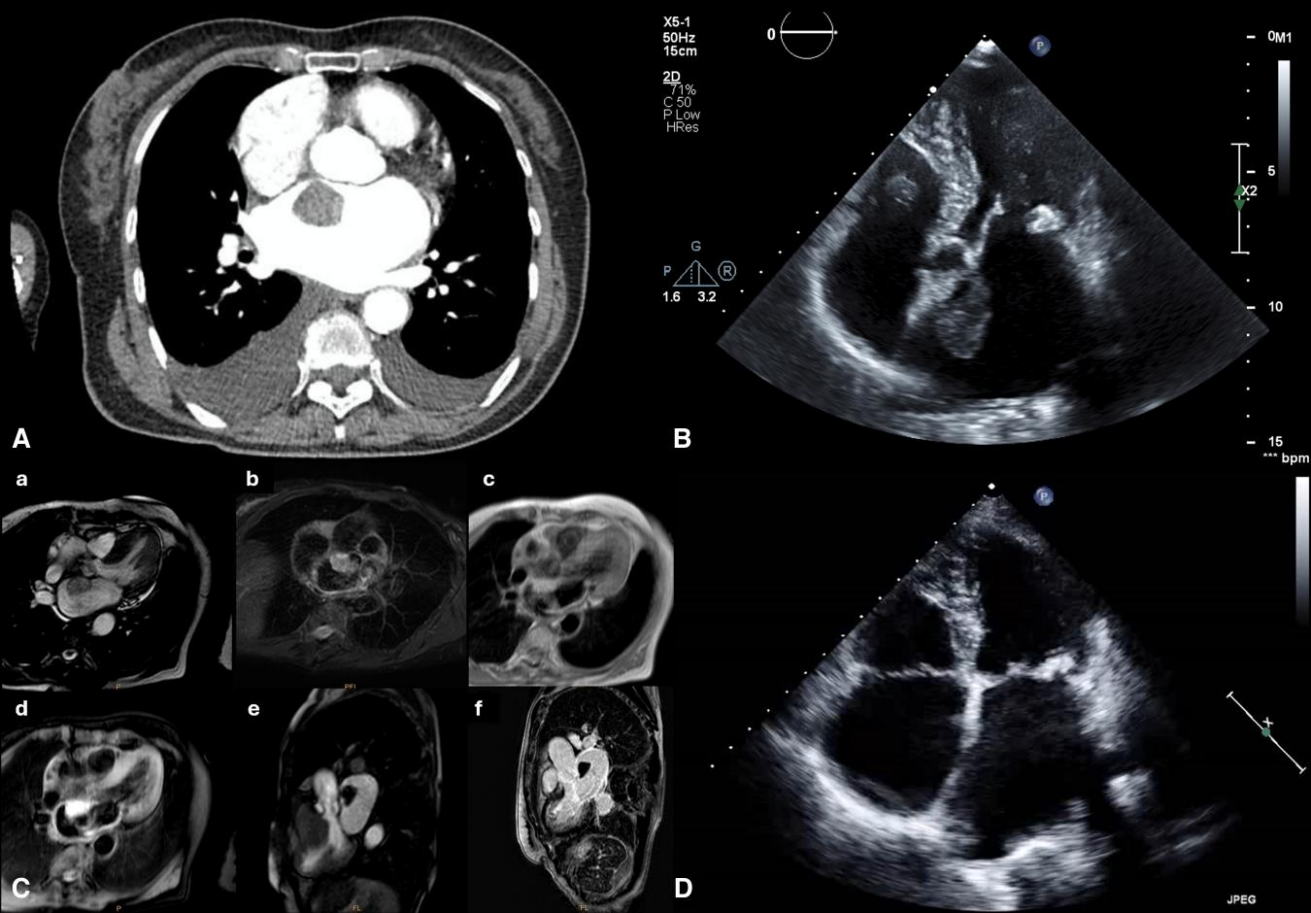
(*A*) Computed tomography (CT) pulmonary angiography demonstrating a large mass in the left atrium; (*B*) Transthoracic echocardiography (5-chamber view) revealing a mobile, heterogeneous mass (30 × 20 mm), attached to the interatrial septum. This image corresponds to Supplementary material online, Videos S1-S2; (*C*) Cardiac magnetic resonance: (C-a) Cine image SSFP sequence of the left atrial mass, (C-b) High-intensity signal on the fluid-sensitive sequence (T2 STIR), (C-c) High-intensity signal on the fluid-sensitive sequence (T1 STIR), (C-d) No signal on the fat-suppressed image; (C-e) No first-pass perfusion, (C-f) No contrast enhancement. This image corresponds to Supplementary material online, *Figure S2*; (*D*) Follow-up transthoracic echocardiography demonstrating complete resolution of the thrombus. SSFP: steady-state free precession, STIR: short time inversion recovery.
